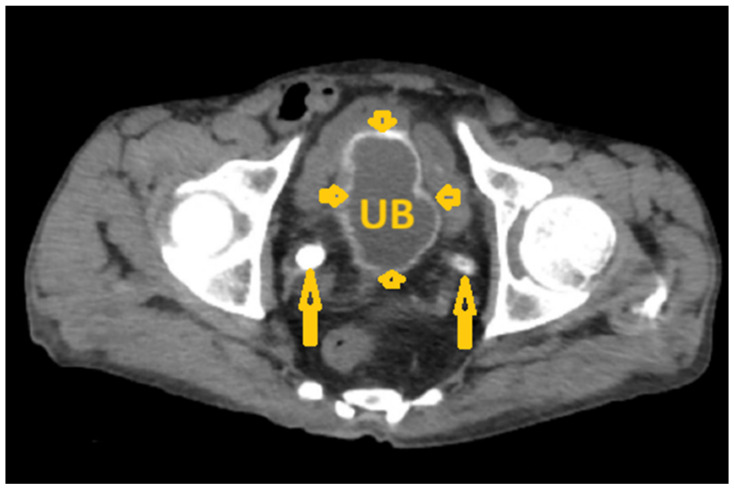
Selected non-contrast computed tomography axial image of a 64-year-old male patient shows diffuse calcification of the urinary bladder wall (short arrows) and bilateral distal ureter calcification (long arrows), reflecting *Schistosoma* cystitis in a proven case of chronic infection with *Schistosoma haematobium*. UB: Urinary bladder.
